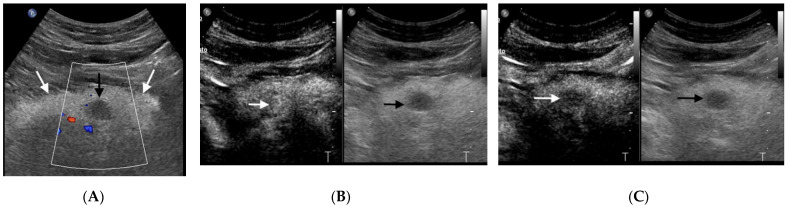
Pancreatic NET: insulinoma. (A) Color Doppler Ultrasonography (white box) depicts lack of vascularity within a 1.5 cm hypoechoic mass (black arrow) in the pancreatic body (white arrows). (B) On CEUS, in the arterial phase (19 s after the injection of c.a.), the mass is slightly hyperechoic in comparison with the adjacent pancreatic parenchyma (arrow), indicating rich vascularity. (C) On CEUS, in the late phase (120 s after the injection of c.a.), the mass is hypoechoic in comparison with the adjacent pancreatic parenchyma (arrow), indicating “wash-out”.
